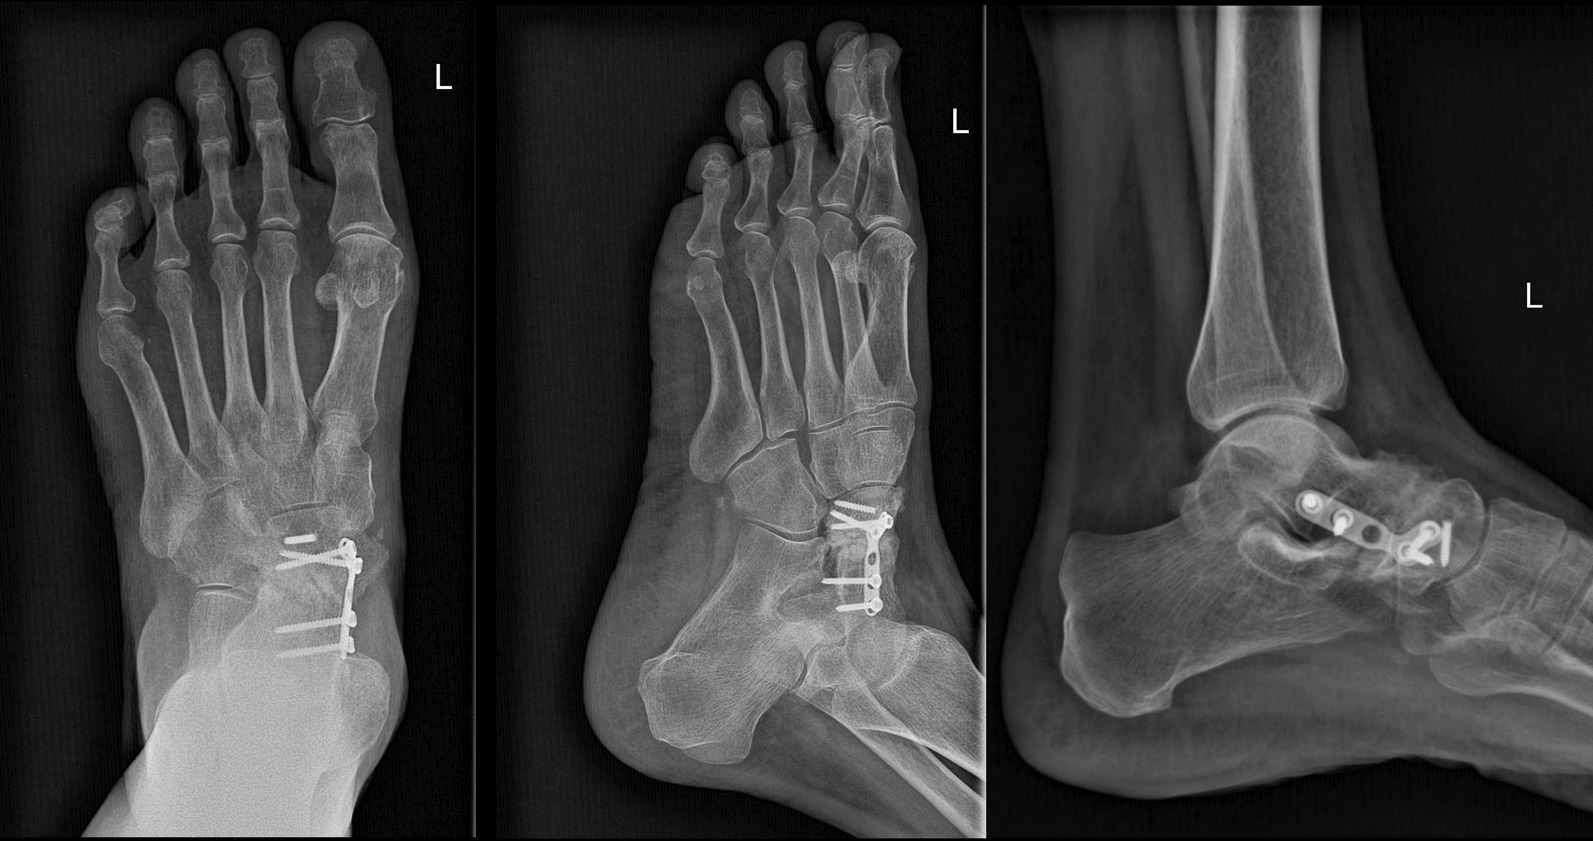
Anterioposterior (AP), lateral, oblique radiography showing union in the talonavicular joint 12 months after the second surgery
